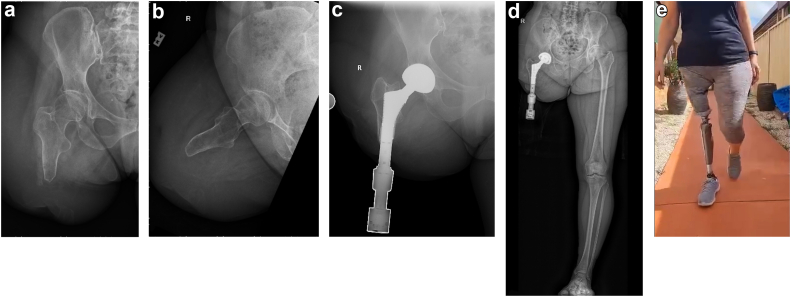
Patient 4 presented as a 53-year-old woman with a proximal right femur amputation performed 41 years prior to manage osteosarcoma. (a, b) Anterior-posterior and lateral radiographs of the right femur identify a residual limb too short to wear a prosthesis, rendering her wheelchair-bound. (c, d) Anterior-posterior right hip and long-standing radiograph taken 5 years after simultaneous THA + TFOI identify the reconstruction; note the femoral component of the THA has a male Morse taper that mates with the female Morse taper of the TFOI implant. (e) A still frame exported from Video 2 taken 6 years after THA + TFOI demonstrates she can walk comfortably in normal tandem gait.
